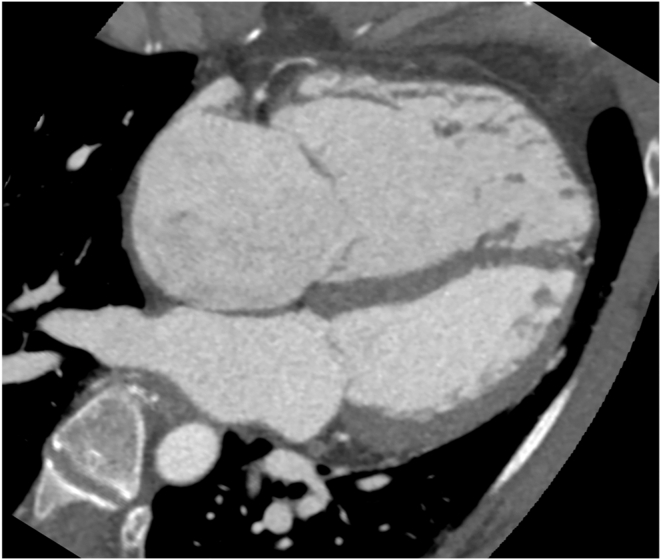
Cardiac computed tomography, oblique axial plane, cardiac 4-chamber view, demonstrates a dilated RV with abnormal septal position consistent with right ventricular volume overload.
